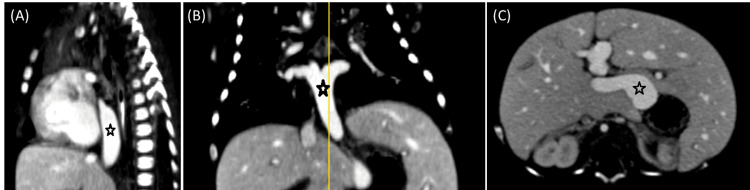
Selected coronal, sagittal, and axial CT scan cuts at the level of the chest (A-B) and upper abdomen (C) show an infracardiac total anomalous of pulmonary venous return communicating with the portal vein (star). CT: Computed tomography
